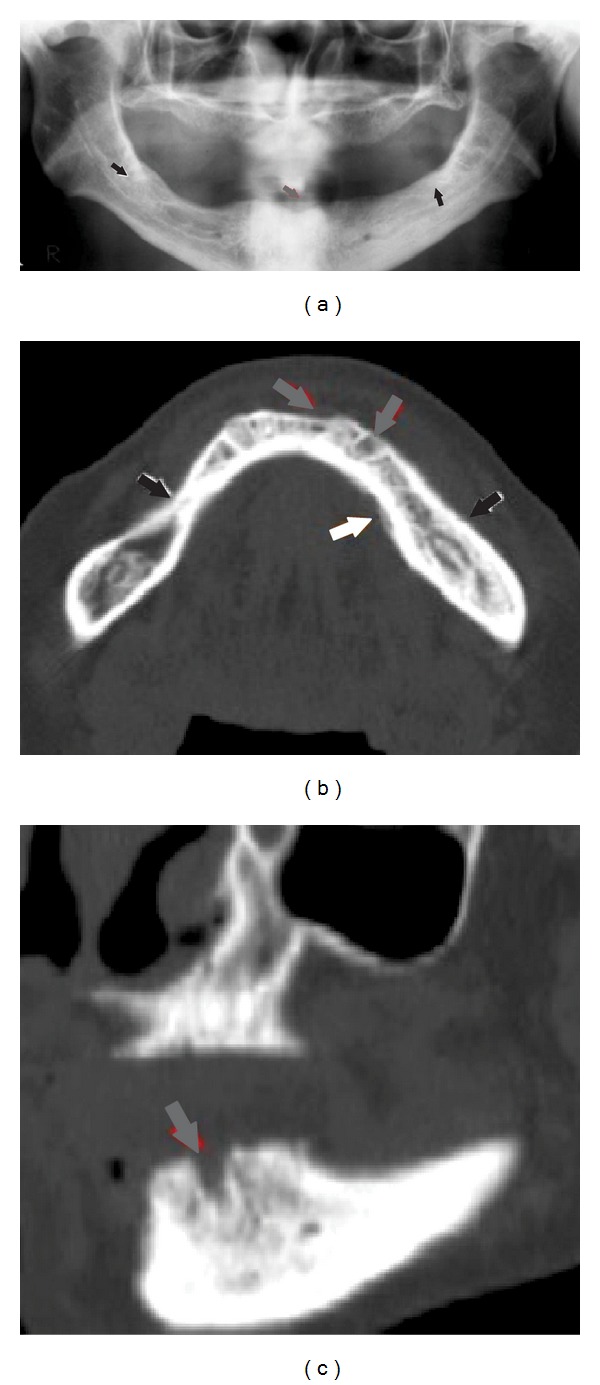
Imaging findings of a 65-year-old woman with metastatic breast carcinoma receiving intravenous zoledronic acid. (a) Panoramic radiograph showing an osteolytic lesion in the anterior mandible (gray arrow) and areas of osteosclerosis in the posterior regions (black arrows). (b) Axial CBCT image reveals areas of osteolysis (gray arrows), areas of osteosclerosis (black arrows), and a periosteal bone reaction in the left mandible (white arrow). (c) Sagittal CBCT image demonstrates a nonhealing extraction socket in the anterior mandible.
